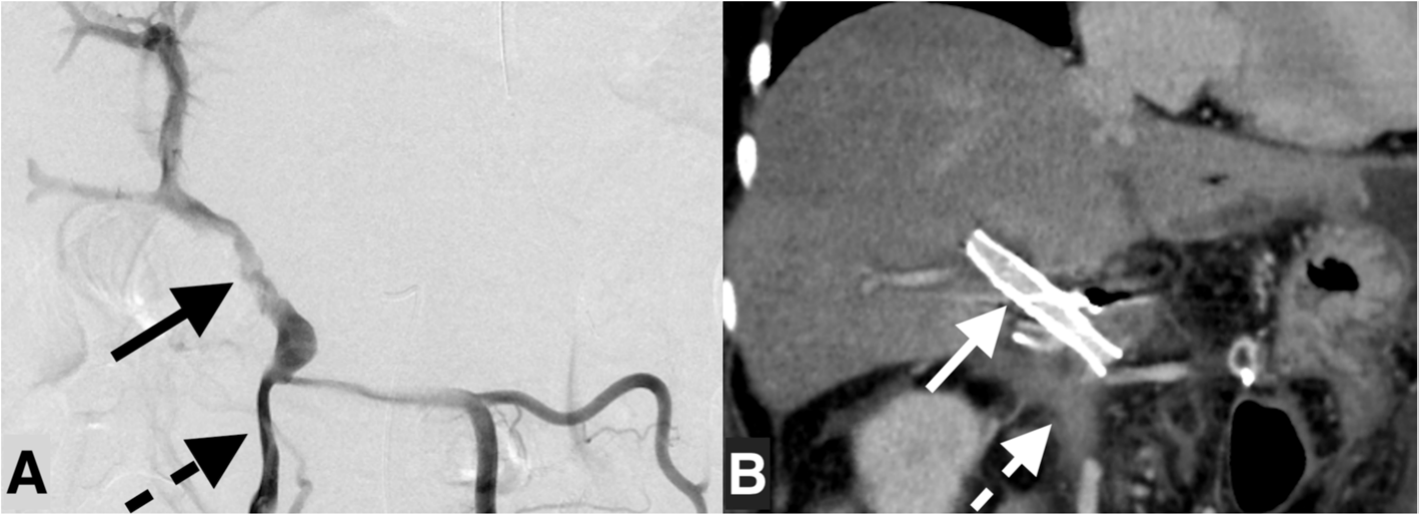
A patient with residual stenosis (outside-stent) which resulted in stent occlusion. A man in his 60 s presented with melena two months after subtotal stomach-preserving pancreatoduodenectomy for ampullary cancer. The contrast enhancement of the portal vein (PV) to superior mesenteric vein (SMV) was unclear and considered to be an occlusion or severe stenosis. Late-onset pancreatic juice leakage was considered as a cause. PV stent placement and total pancreatectomy (to control pancreatic juice leakage) was planned emergently. A Portography shows PV stenosis (arrow). Two stents (SMART Control; Cordis, Hialeah, FL, USA) were placed through the PV and post-stent balloon dilatation was performed up to the nominal pressure. Stent placement was not performed for SMV because it was unrecognized (dotted arrow). After the stent placement, PV flow improved. Subsequently, residual total pancreatectomy was performed. B Contrast-enhanced CT six days after the stent placement (arrow). The contrast enhancement of the SMV on the caudal side of the stent is unclear (dotted arrow). Two years and three months after placement, the stent occluded
